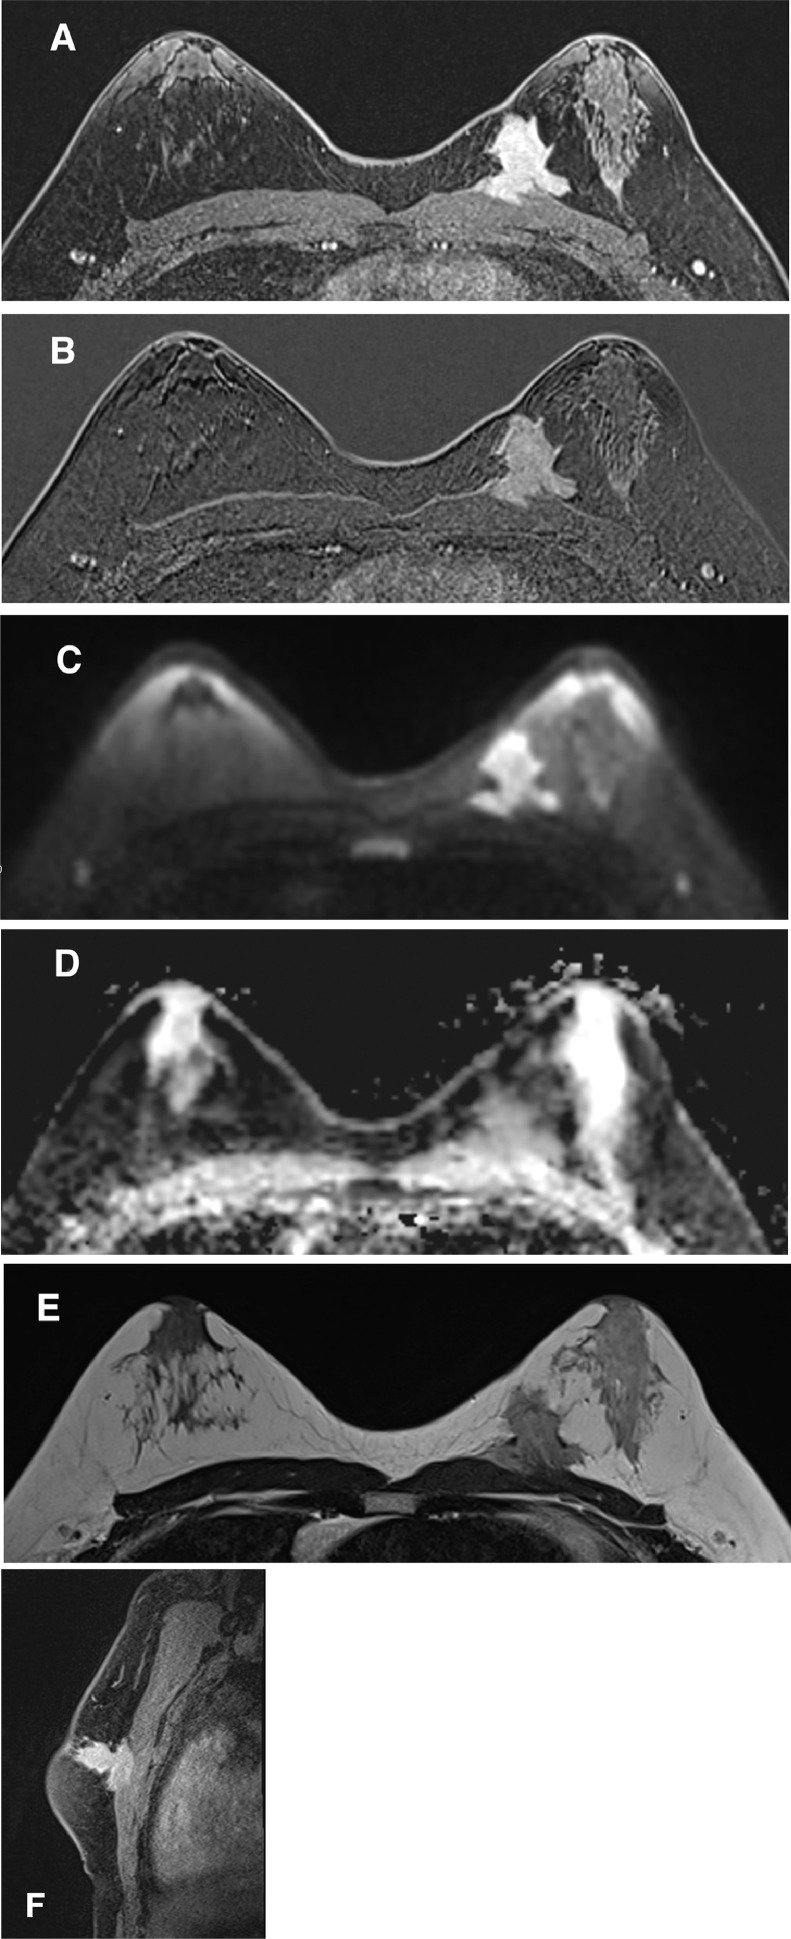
MRI (A) Dynamic T1-weighted axial sequence. (B) Dynamic T1-weighted axial sequence with subtraction. (C) Axial diffusion sequence. (D) ADC axial sequence. (E) Axial T2-weighted sequence. (F) Late T2-weighted sagittal sequence. Depicts a spiculated mass in the upper quadrant at 10-o'clock of the left breast, demonstrating enhancement after contrast injection, accompanied by skin retraction and infiltration of the pectoralis major muscle. Additionally, diffusion restriction and late enhancement are observed.
